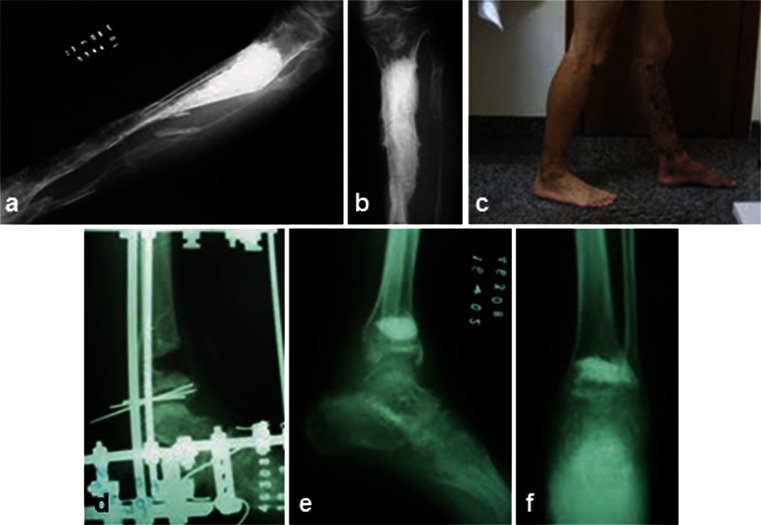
Reconstruction of tibial defects caused by tumors (a–c) and traumatic defects (d–f) using granules of BCP corresponding to 20-40 mesh. Postoperative x-rays of the tibia (a, b) and clinical aspect of the patient (c). Preoperative image of the bone defect (d) and its postoperative analysis in the lateral (e) and antero-posterior view (f) (provided by Dr. W.N. da Silva)
